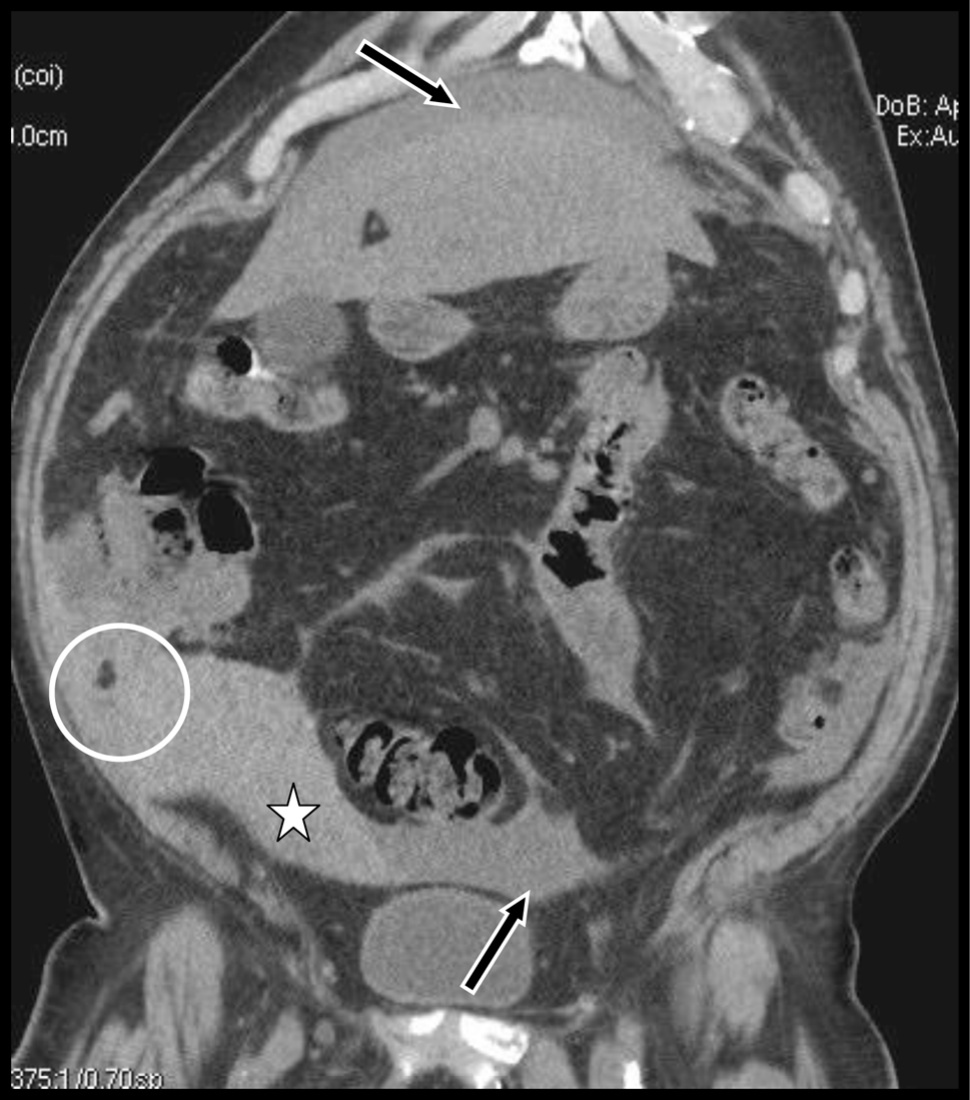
Coronal view of unenhanced abdominal CT shows diffuse ascites (black arrows) that appears spontaneously dense in the hypogastric area and in the right iliac fossa (white star) evocating hemoperitoneum. A small lipomatous masse is trapped in blood in the right iliac fossa (white circle).
